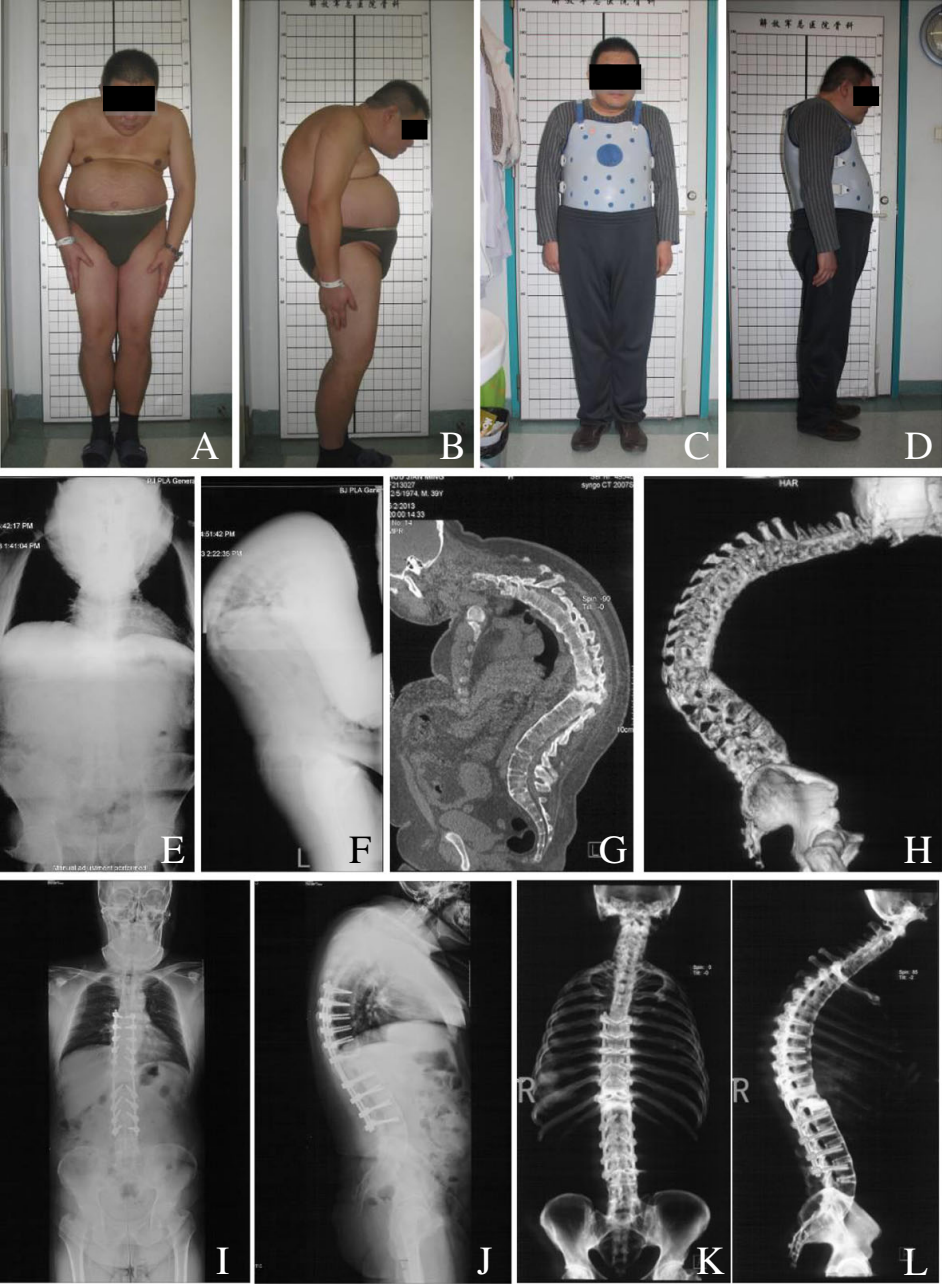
A 39-year-old male patient suffering from Andersson lesion-complicating ankylosing spondylitis. a–d Clinical appearance before surgery, and the final follow-up of 2 years. e, f Preoperative radiograph showed a remarkable kyphosis in thoracolumbar spine with a regional kyphosis of 72. g, h CT sagittal reconstruction image demonstrated fractures with irregular discovertebral osteolysis. i, j The X-ray at 2 years postoperatively showed the regional kyphosis was corrected to 17. k, l Three-dimensional reconstruction demonstrated that solid fusion of resection site was achieved
